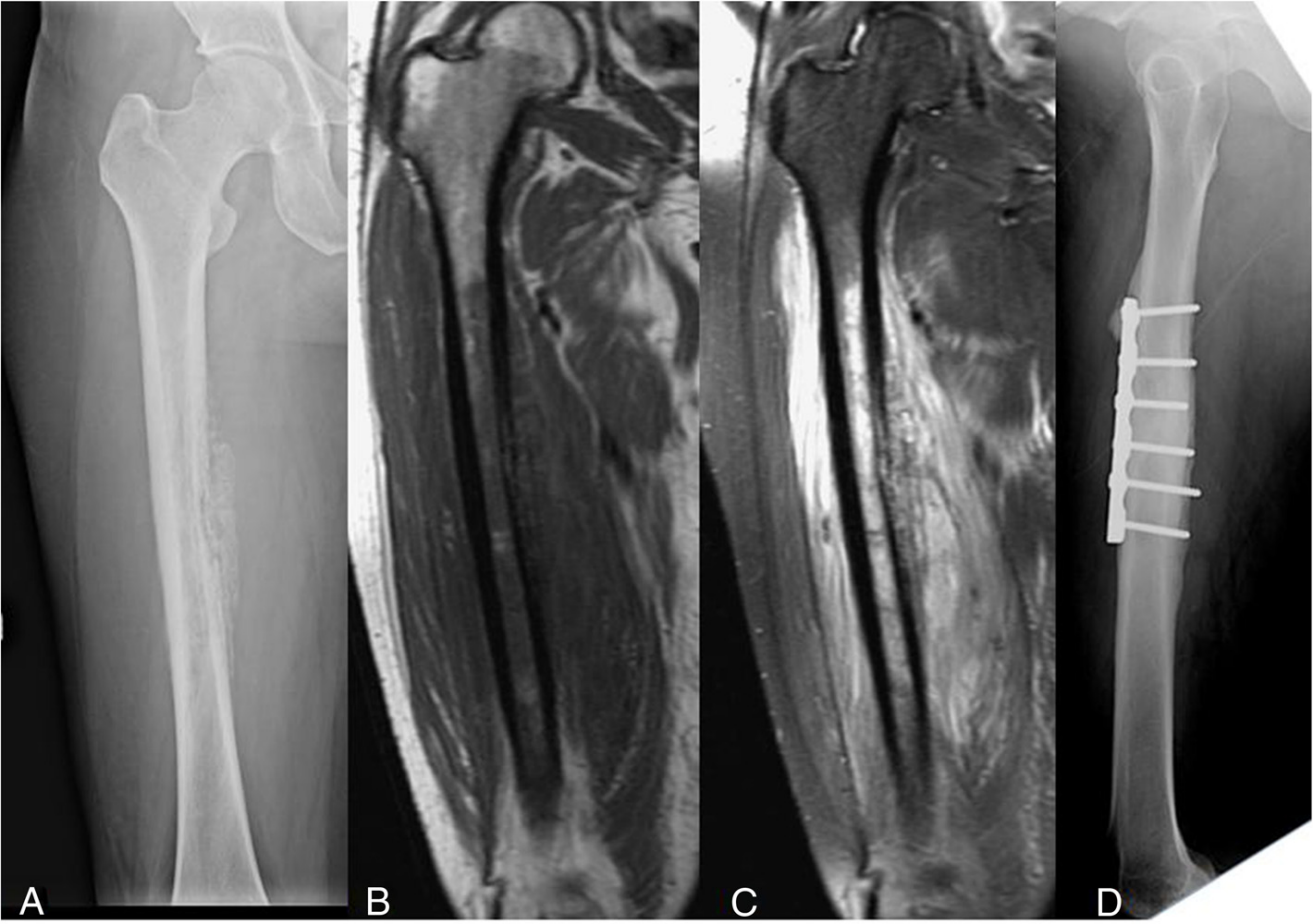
A 54-year-old woman with a history of type 2 diabetes mellitus who complained of moderate nocturnal thigh pain for three months. (A) A plain film of the femur showing a laminated periosteal reaction with partial cortical destruction and moth-eaten osteolytic lesions in the medulla. (B, C) T1- and T2-weighted magnetic resonance imaging (MRI) showing cortical destruction with extensive soft tissue edema. (D) A plain film taken 18 months after debridement and open reduction and internal fixation (ORIF) surgery showing bony healing with no signs of recurrence.
